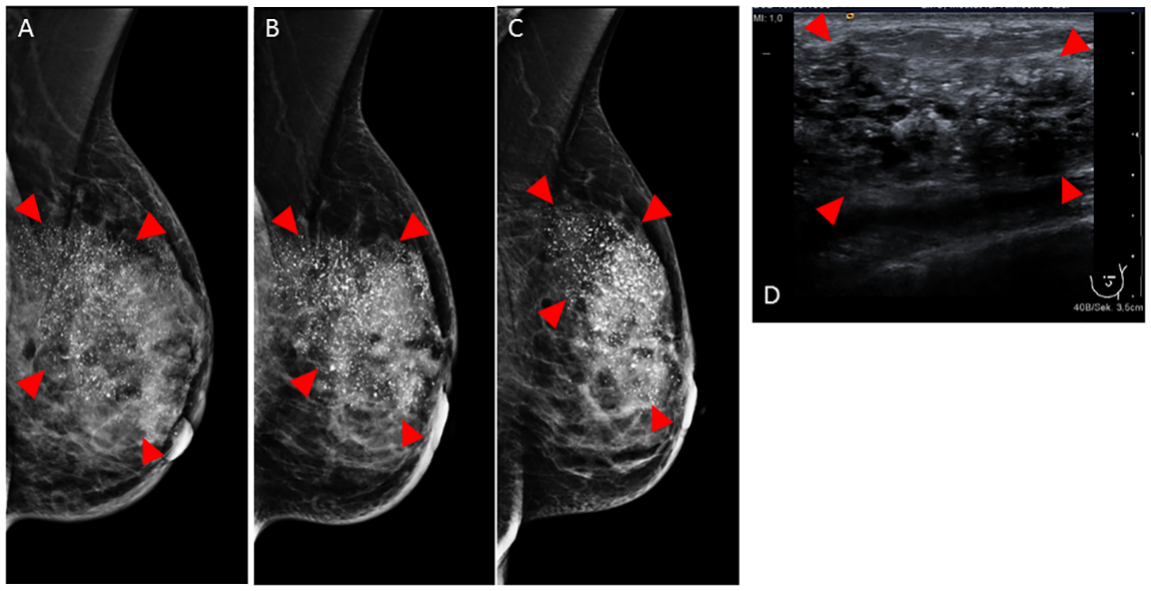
Clinical mammography and sonography of patient B. In-vivo mammography in mediolateral oblique projection before (A), after 4 cycles NAC (B) and after completion of NAC (C). Ultrasound before NAC (D). Tumor indicated by arrowheads. Please note the excessive calcifications (white spots in A–D) within the tumor. The exact tumor borders are not visible in mammography and sonography.
